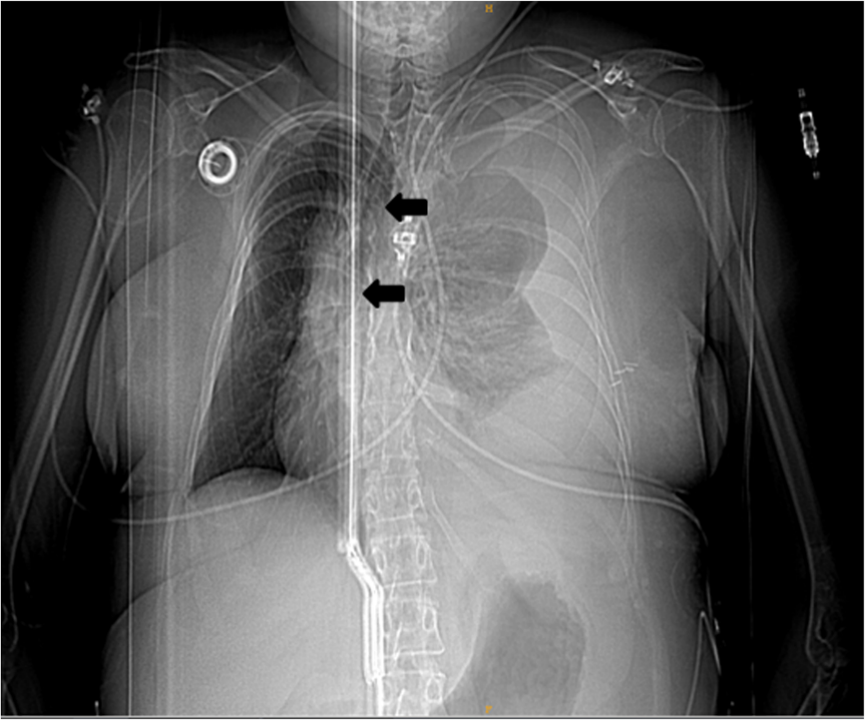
Chest radiography showing extensive opacity in the left hemithorax. Note the massive mediastinal shift (arrows).
